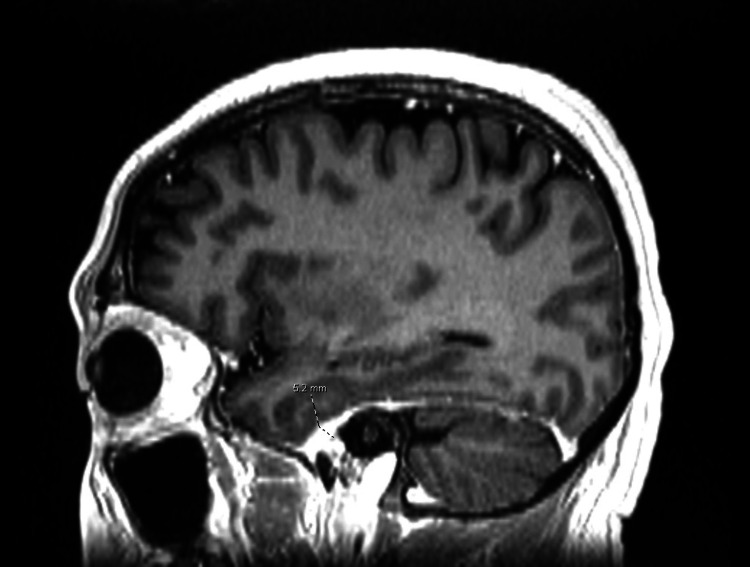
Sagittal T1-weighted MRI scan of the head showing 5.2 mm smooth dural thickening and enhancement anteriorly (dotted label) consistent with IHP, as opposed to GPA which typically displays local bone destruction at the base of the skull and vascular inflammation. MRI: Magnetic resonance imaging; IHP: Idiopathic hypertrophic pachymeningitis; GPA: Granulomatosis with polyangiitis
